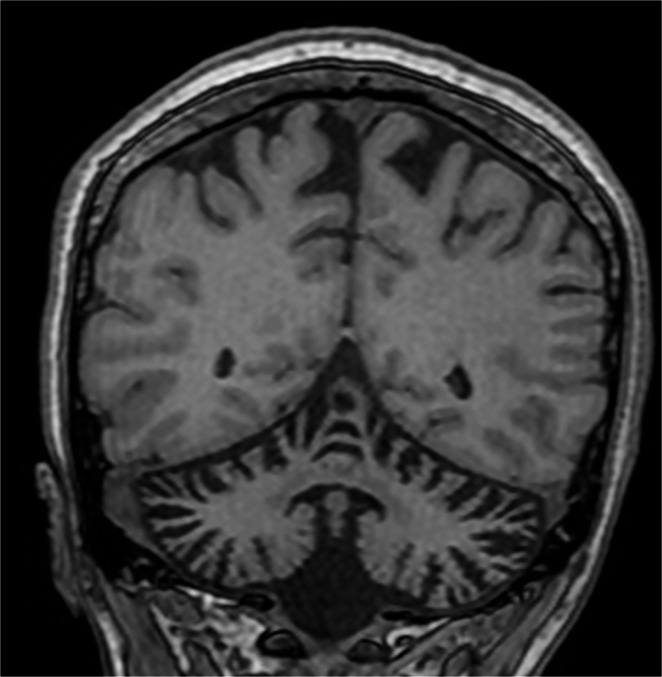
Coronal T1-weighted brain MRI demonstrates marked global cerebellar atrophy
